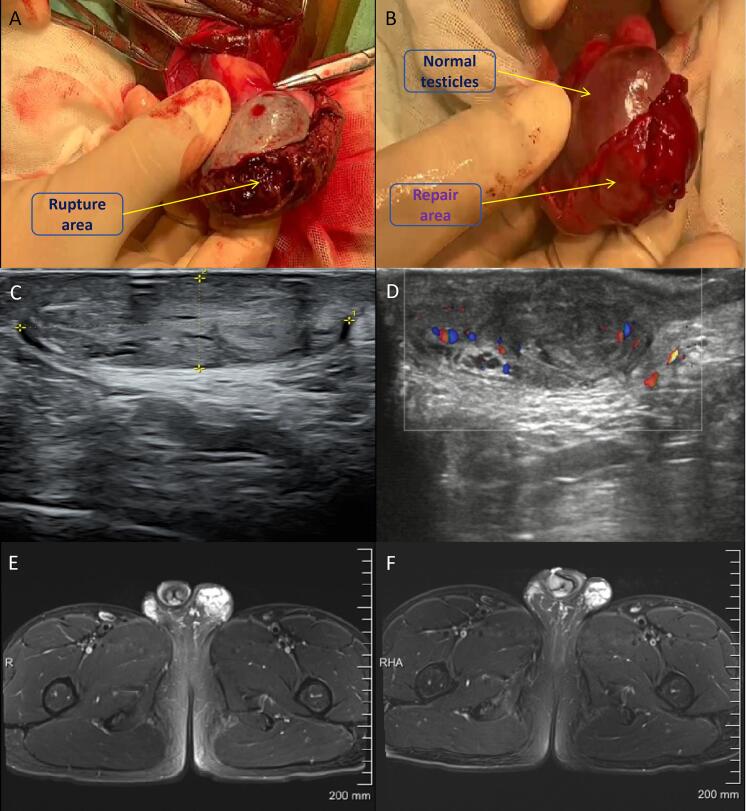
(A) During the operation, a large area of tunica albuginea defect and testicular parenchyma protrusion were observed in the testicles. (B) Repair the tunica albuginea defect with tunica vaginalis, with a length of approximately 4 cm. (C) Six months after surgery, B-ultrasound showed that the testicular morphology was still acceptable. (D) Six months after surgery, B-ultrasound showed good blood supply to the testicular repair site. (E) Three months after surgery, MRI showed good testicular recovery. (F) Six months after surgery, MRI showed a reduction in testicular volume.
